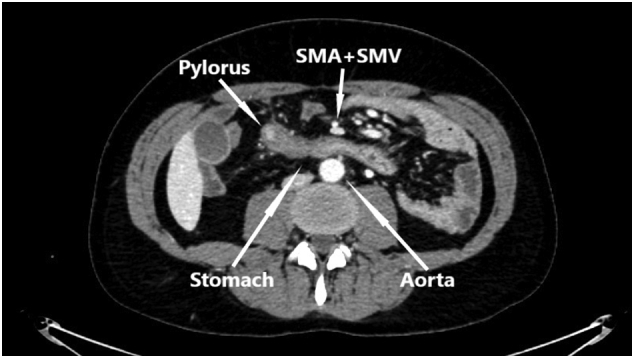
Preoperative contrast enhanced CT study image. Displacement of superior mesenteric artery (SMA) and superior mesenteric vein (SMV) anteriorly to stomach.
